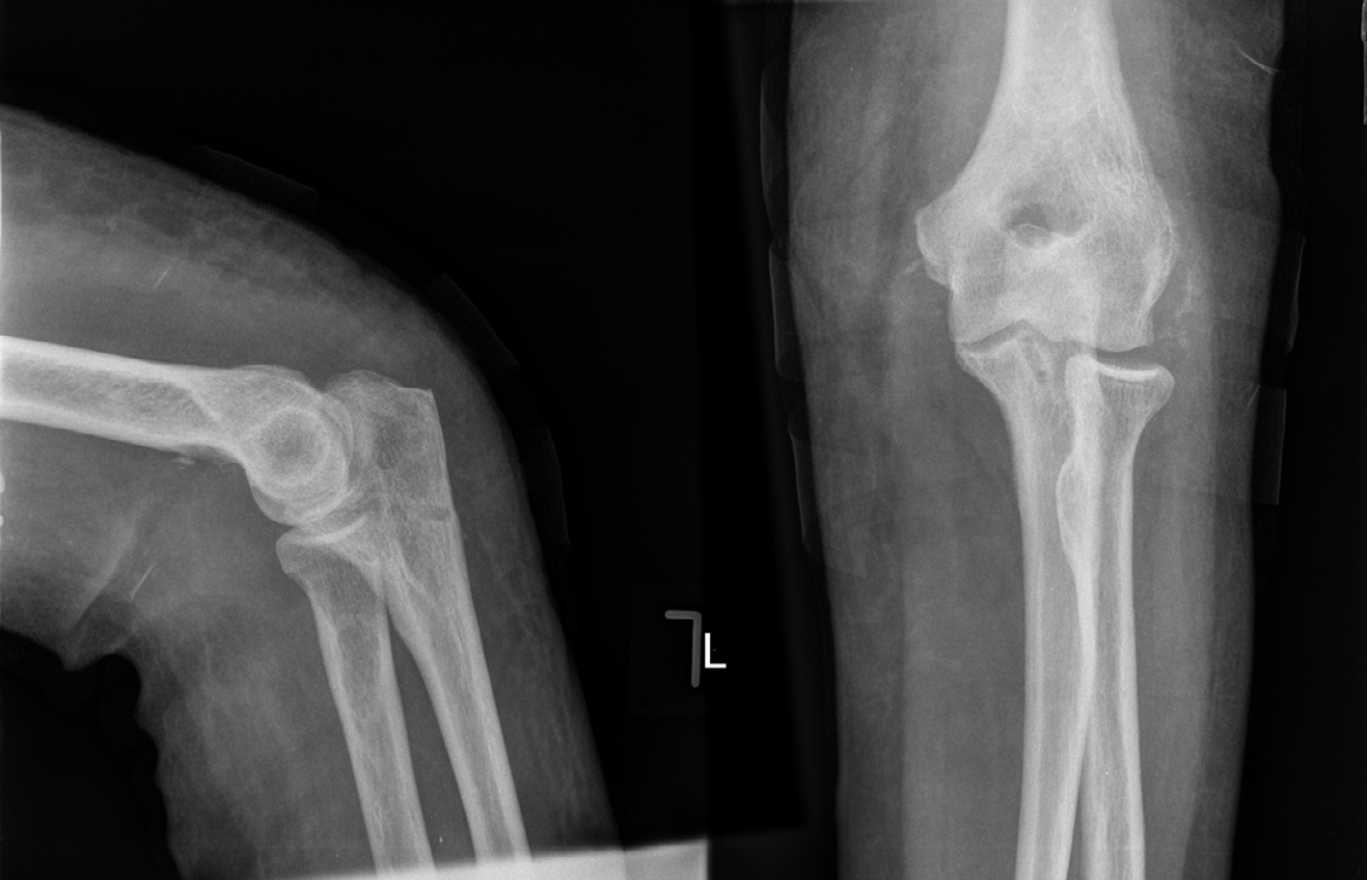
Elbow AP and lateral radiographs two weeks post initial reconstruction.
